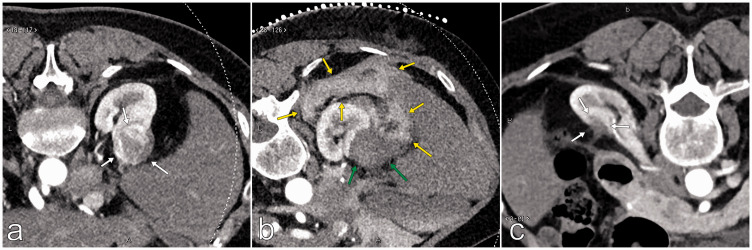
Example of a completely ablated renal tumor (clear-cell carcinoma) with MWA. (a) Preprocedural CE-CT showed a 33-mm tumor, m-RNS 10a (white arrows). (b) Hydrodissection was used to increase the distance between the liver and renal tumor (yellow arrows). After a total ablation time of 12 min, immediate CE-CT control showed a completely ablated tumor (green arrow). (c) At the six-month follow-up, CE-CT showed complete tumor treatment, without contrast enhancement in the treated area (white arrows). CE-CT, contrast-enhanced computed tomography; m-RNS, modified RENAL nephrometry score; MWA, microwave ablation.
